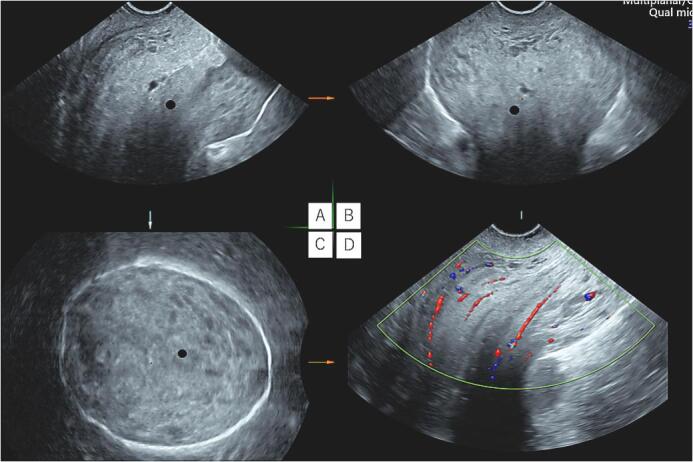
Cervical edema and cervical length of 8.8 cm, color Doppler with 3D transvaginal ultrasonography. A, Sagittal section; B, Coronal section; C, Transverse section; D, Color Doppler.
